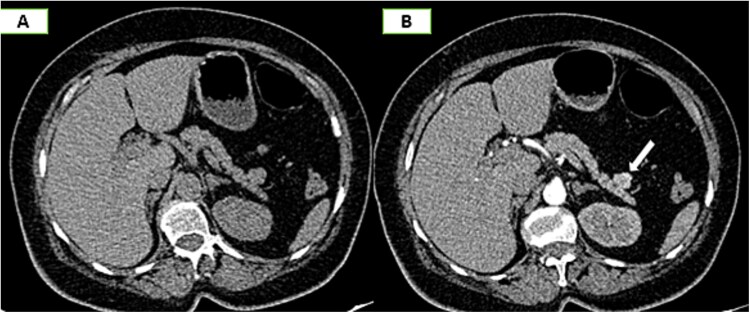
Axial sections of an abdominal non-contrast CT scan (A) and in the arterial phase (B) reveal a nodule adjacent to the tail of the pancreas that is hypervascular on arterial phase and follows the same pattern of enhancement as the spleen.
